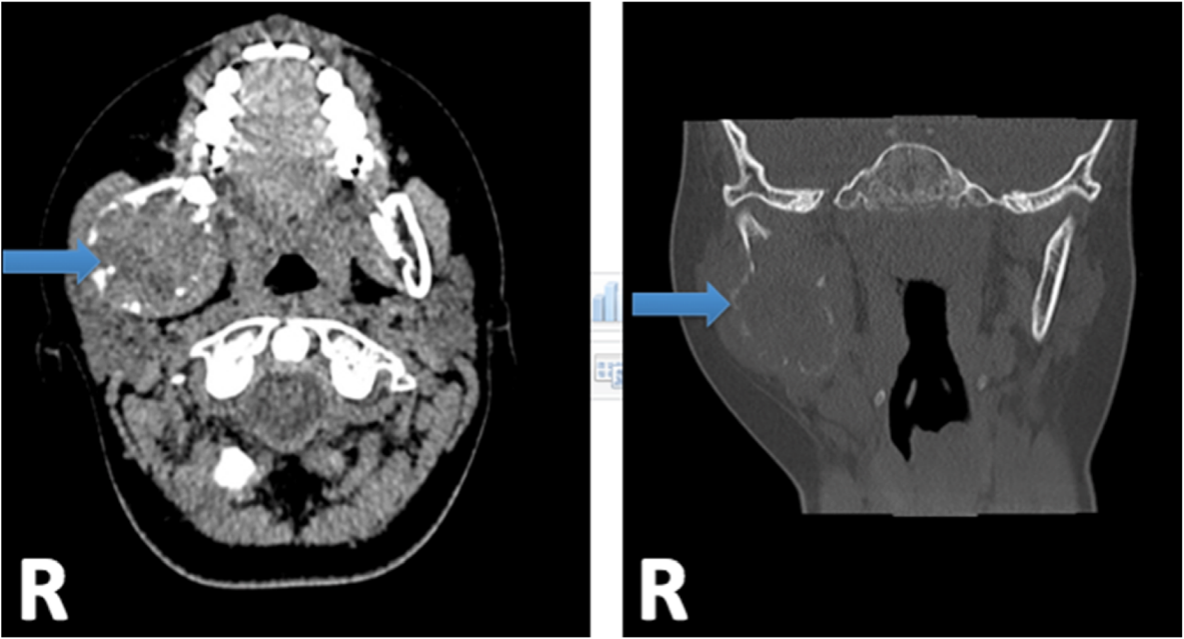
CT Head following initial presentation to the emergency department, with axial and coronal views. Arrows are pointing to site of lesion
